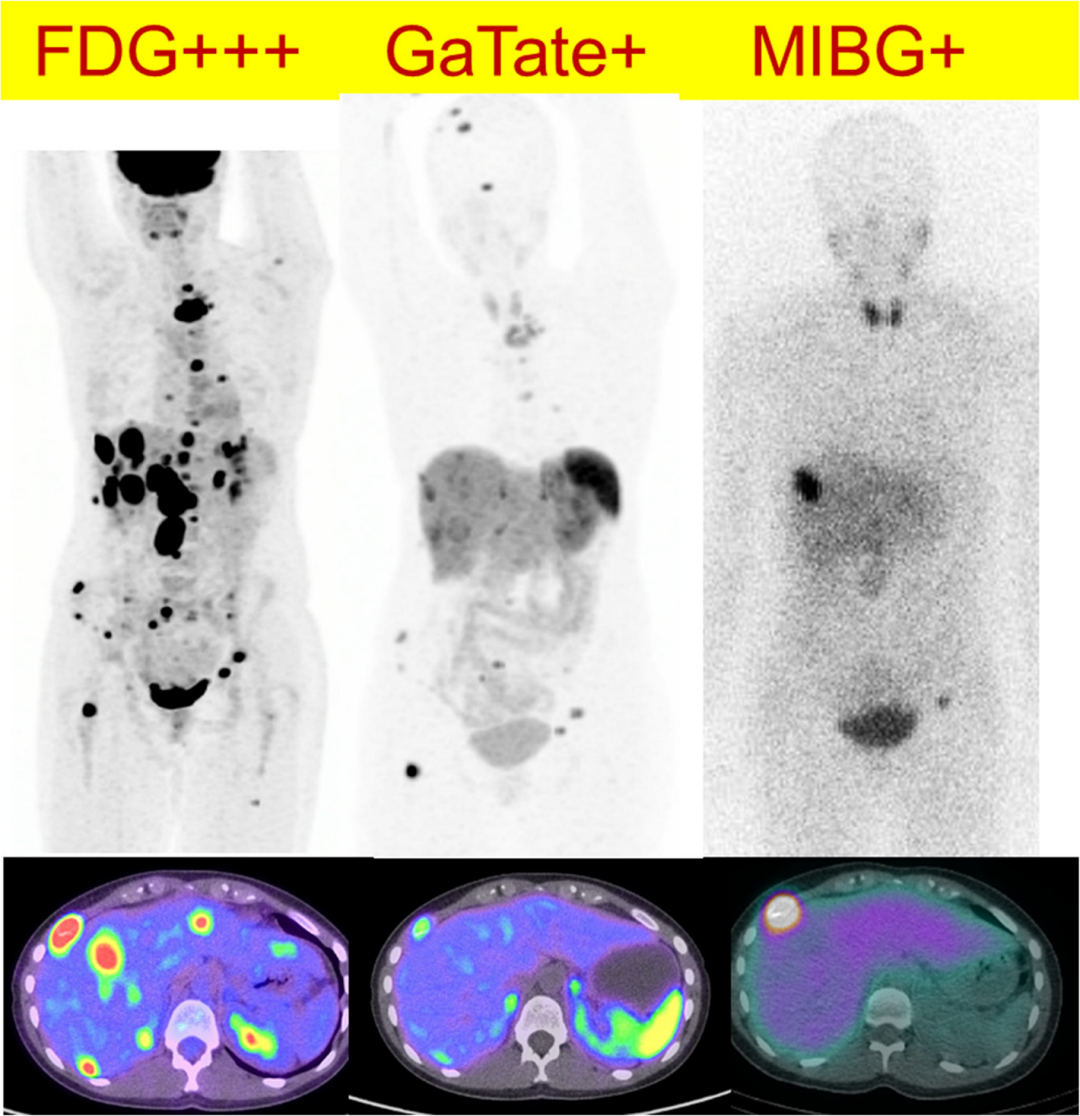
A 25 year old female with *SDHB* germline mutation and metastatic PGL. Sites of disease show very high F-18 FDG uptake (*left*) with relative low Ga-68 DOTATATE uptake (*middle*) and MIBG (*right*). The findings indicated lack of suitability for either radionuclide therapy with radiolabelled DOTATATE or MIBG. The patient had a rapidly progressive disease course despite treatment with chemotherapy (combination of cyclophosphamide, vincristine, dacarbazine) and subsequently sunitinib. The lack high SSTR expression is unusual for a *SDHB* phenotype and was indicative of de-differentiated disease with consequent aggressive phenotype
